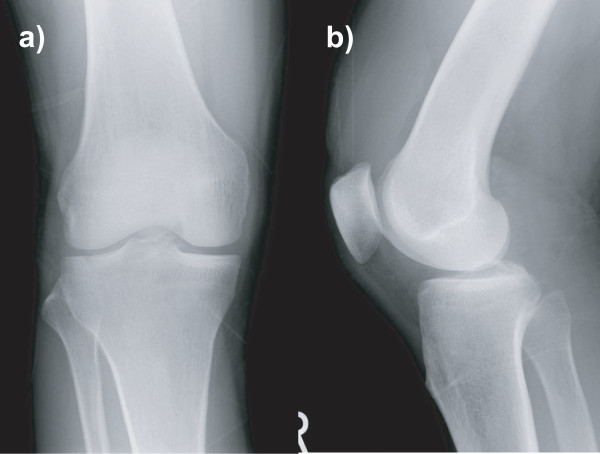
Initial anteroposterior and lateral radiographs of the knee, showing fractures of the lateral tibial condyle (a) and the posterior intercondylar prominence (b).
